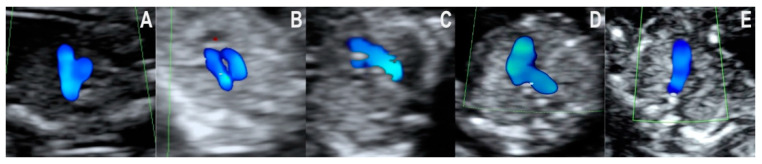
Color doppler flow patterns encountered in the three-vessel and trachea view. (A): Typical V sign with equally sized arms. (B): The vessels forming a U sign around the trachea. (C): V sign with a narrower arm. (D): One vessel with an oblique and curved course. (E): One vessel with a straight course.
